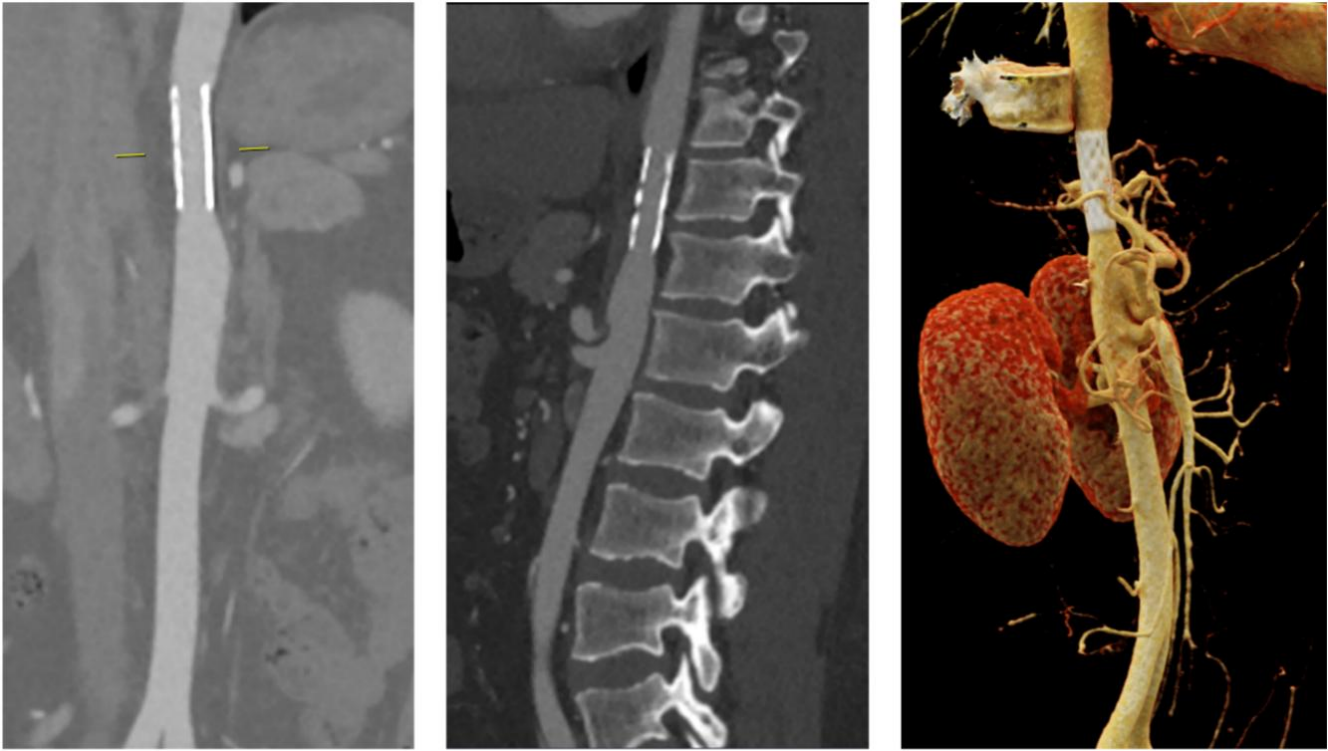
Computed tomographic angiography of the abdominal aorta. Coronal view (left), sagittal view (middle), and 3D reconstruction (right).
